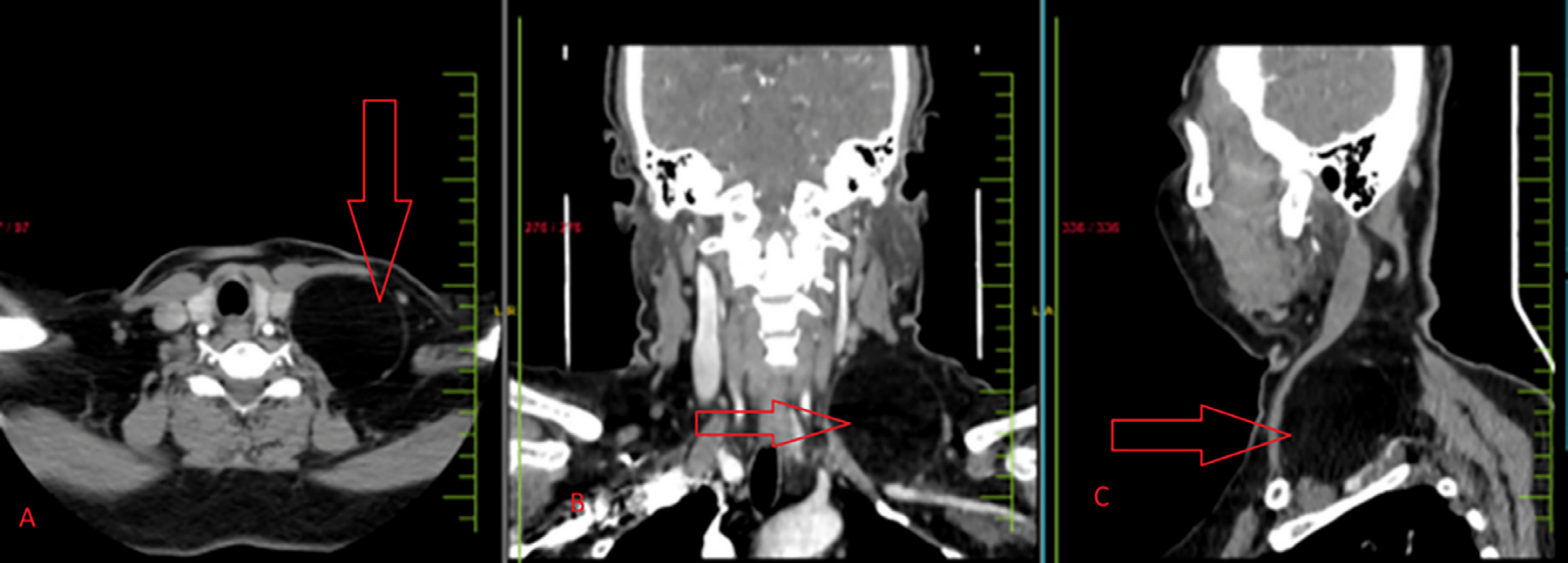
Contrast enhanced computed tomography (CECT) in arterial phase - axial section (A), coronal section (B), sagittal section (C) showing well defined non enhancing hypodense fat density mass lesion in left posterior cervical region, measuring approximately 5.6 × 5 × 6 cm with few internal septation (red arrows). It is seen abutting left sternocleidomastoid muscle and left internal jugular vein.
